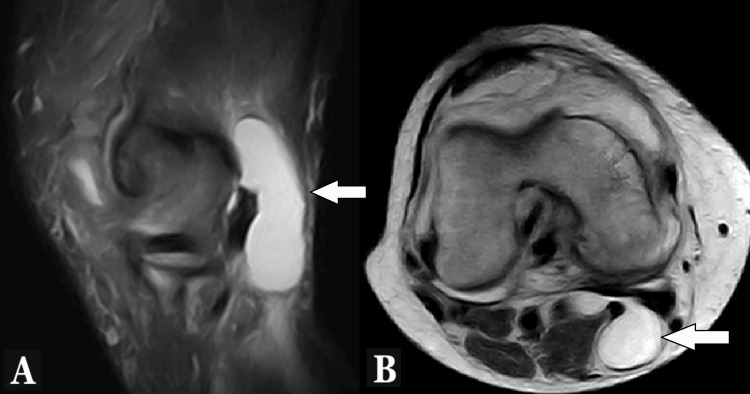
MRI of a Baker’s cyst. MRI shows synovial-fluid distension of the gastrocnemius-semimembranosus bursa, typically maintaining a communication with the knee joint via a posterior capsular opening on the sagittal view (A) and the axial view (B).
